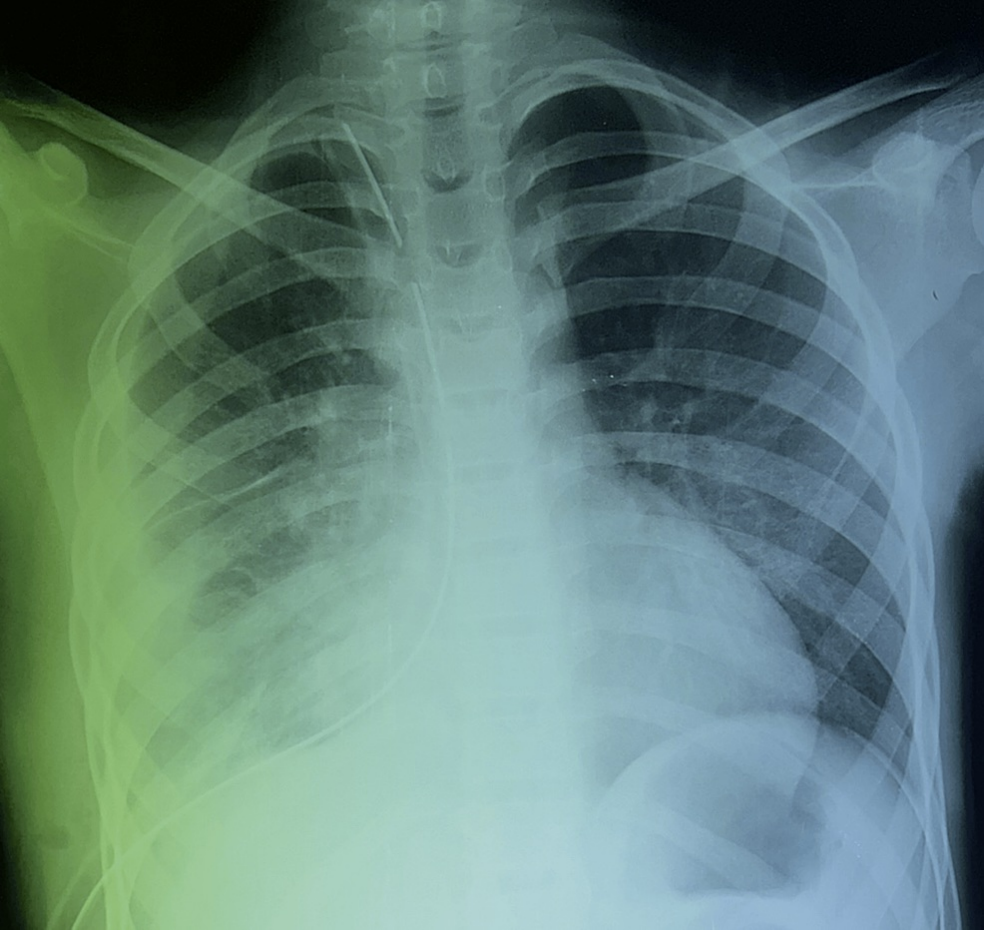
Posteroanterior chest radiograph after pleural decortication The image shows the return of the lung to the wall; mediastinal elements and drains are in place.
